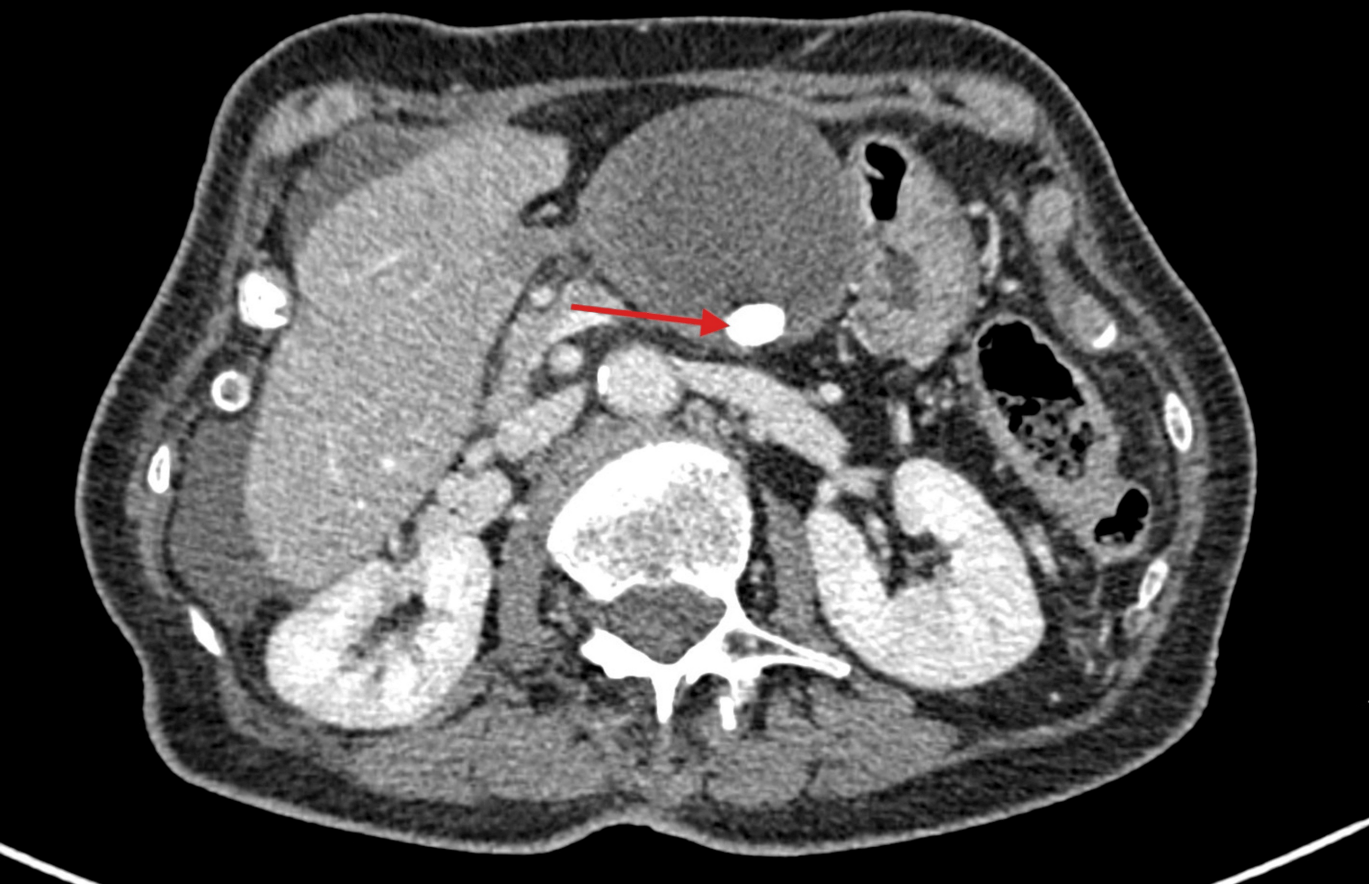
CT scan of the abdomen and pelvis revealed a grossly distended gallbladder with a 14-mm gallstone (red arrow).
